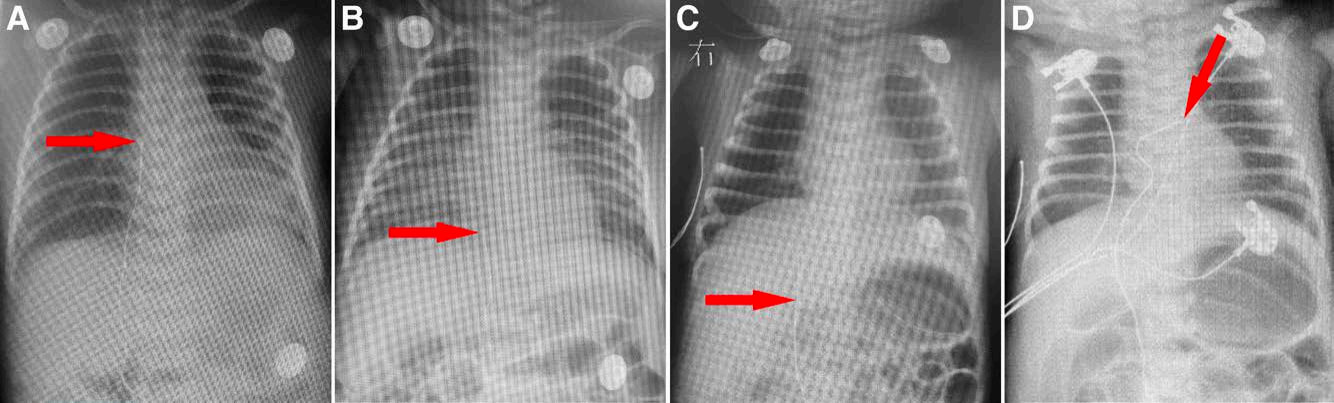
Positioning of catheter tip by chest radiography following peripherally inserted central catheter (PICC) placement, the locations of catheter tip were indicated by the red arrows. (A) PICC tip was positioned at the level of the pulmonary artery, approximately 30 mm from thoracic vertebra (T) 9 at postnatal day (PND) 2 in case 1; (B) PICC tip was located to the right side of T 9 at PND 6 in case 1; (C) PICC tip was positioned at the level of lumbar vertebra (L) 1 at PND 2 in case 2; (D) the PICC catheter traversed the vertebral column at the right side of T 6 and terminated to the left of T 5 at PND 3 in case 2.
